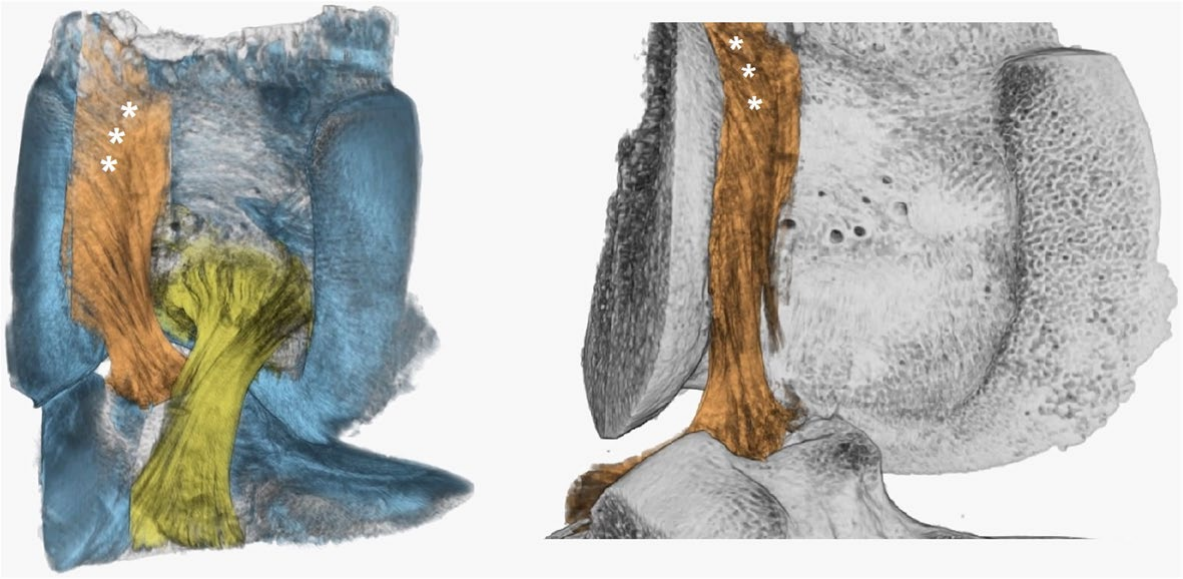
3D- CT of the intercondylar groove. The ACL (orange) is composed of a multitude of fibers. The stars indicate the direct fibers, directed in the “Over the top” position
